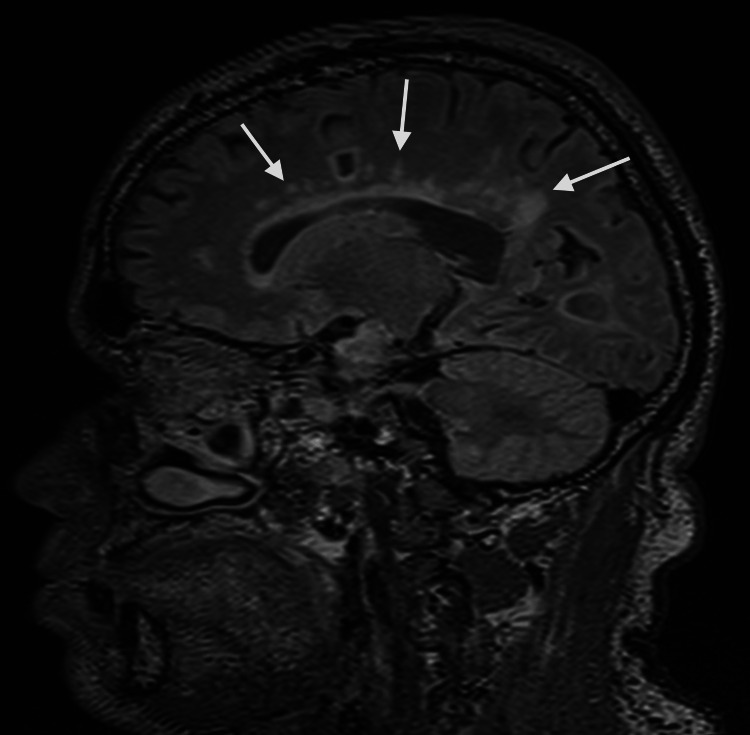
Brain MRI, FLAIR, sagittal view
